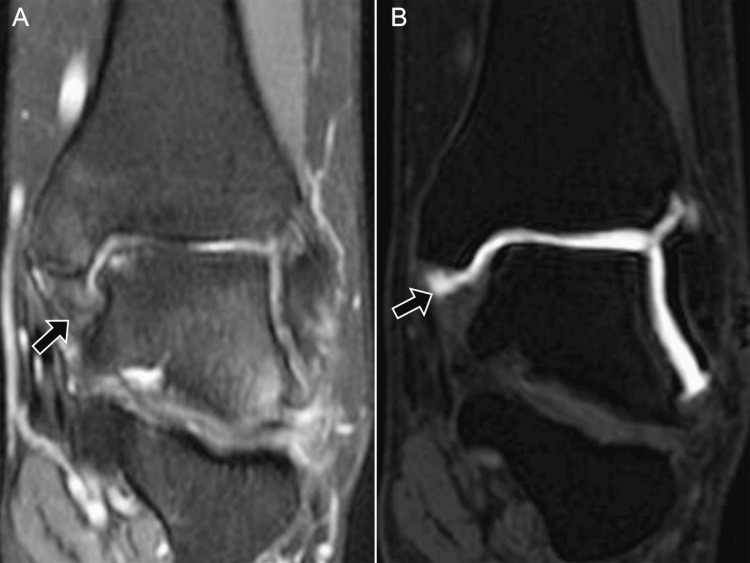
MR arthrography revealing deltoid ligament tear. A 40-year-old male: (A) coronal non-contrast T2 fat-saturated MRI shows a thickened, hyperintense deltoid ligament (arrow); (B) coronal post-contrast T1 fat-saturated MR arthrography reveals a linear hyperintensity indicating a full-thickness tear of the deep deltoid fibers with contrast extension (arrow). Images belong to the authors; patient consent was obtained.
